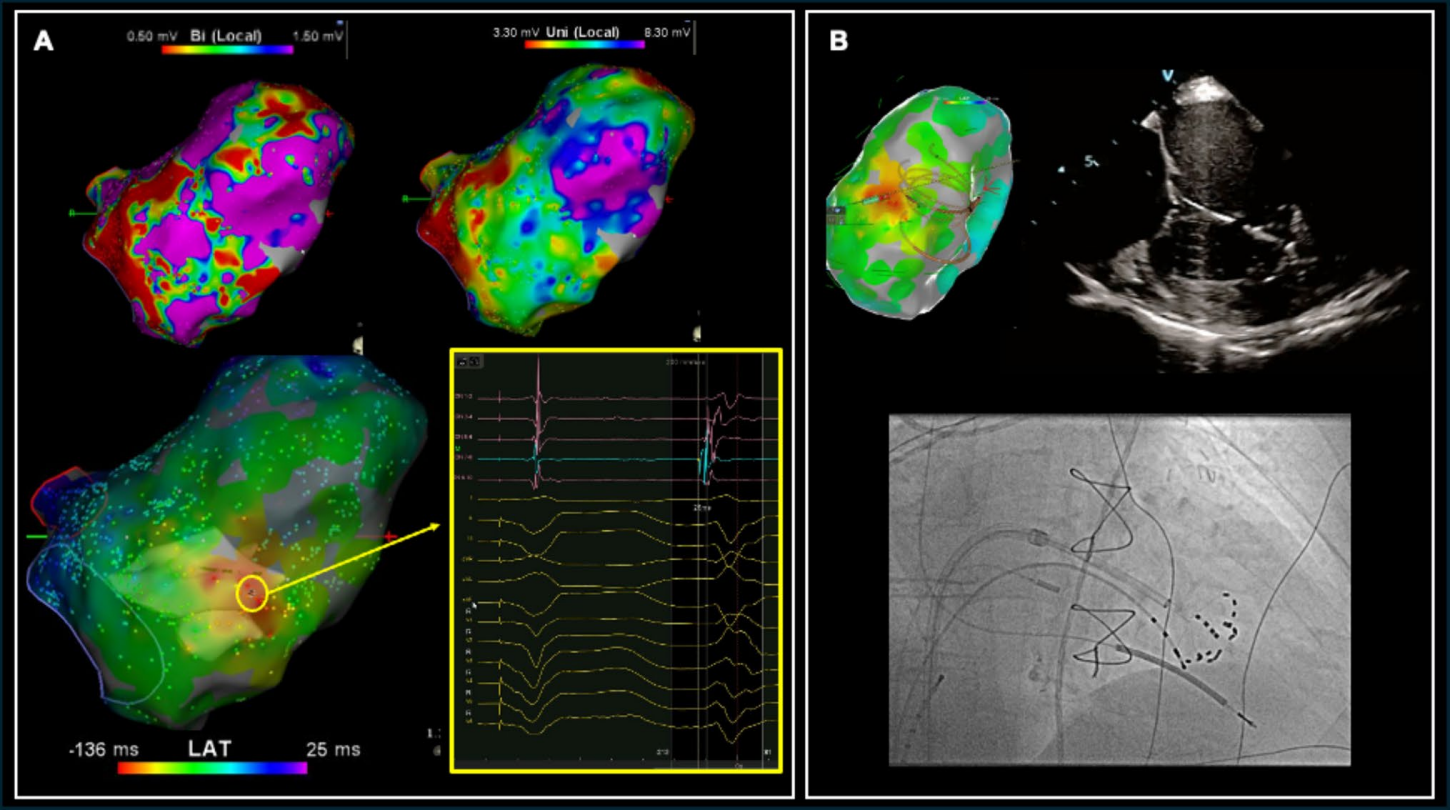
Case 3: A Bipolar and unipolar voltage maps demonstrate post-infarction scarring of the left ventricle. The activation map (made transparent to show the papillary muscles delineated by intracardiac echocardiography) shows earliest site of activation at the base of the posteromedial papillary muscle. The site of earliest activation − 26 ms pre QRS shows a multicomponent fractionated electrogram without clear isolated Purkinje potential at the base of the posteromedial papillary muscle. B Electroanatomic map shows the location of the PFA catheter during lesion delivery, also shown on intracardiac echocardiography where the catheter can be seen with good contact with the papillary muscles. The fluoroscopy image shows flexion of the catheter to aid contact with the posteromedial papillary muscle
